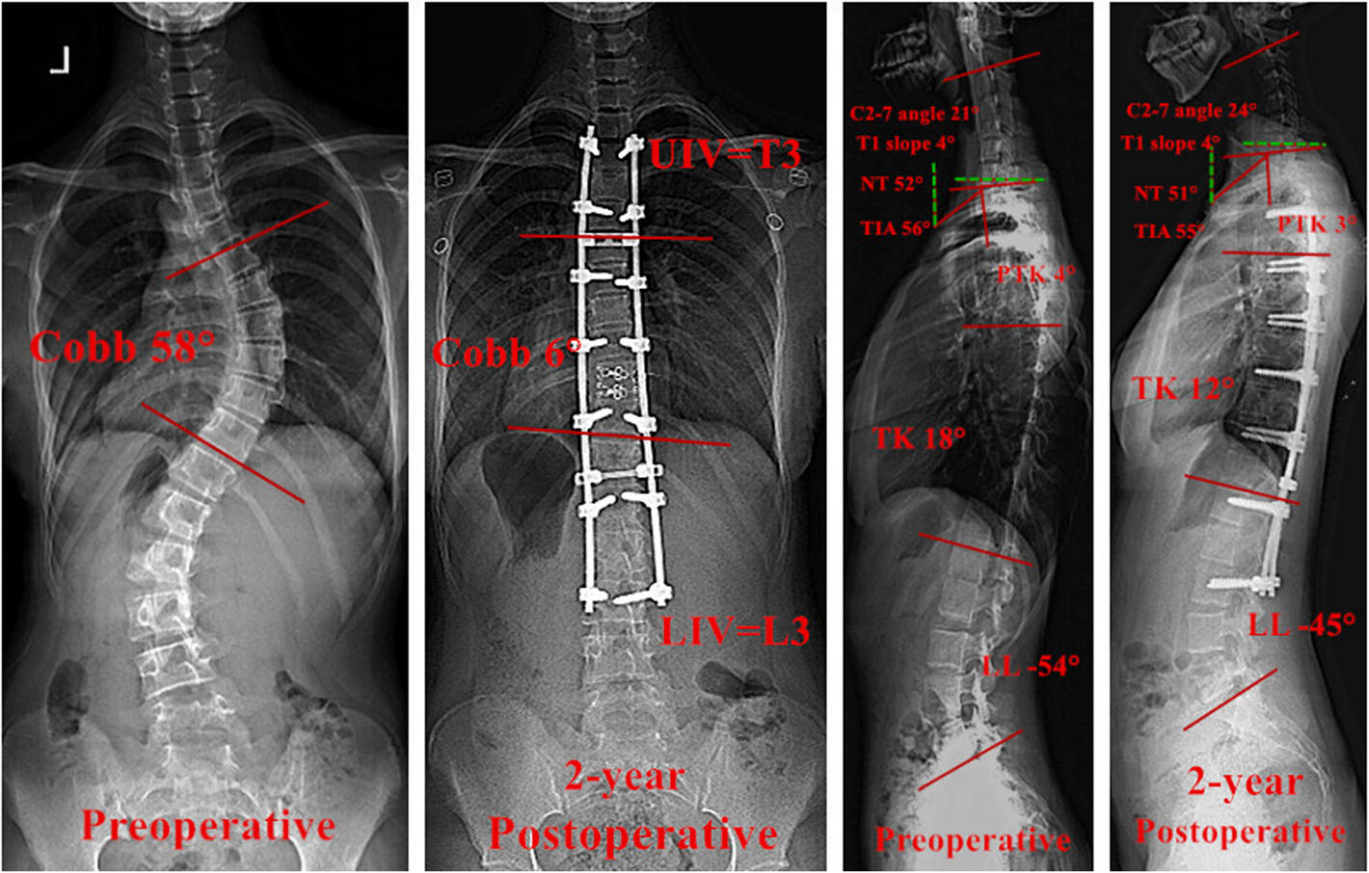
The pre- and 2-year postoperative radiographs of a Lenke 1 patient with AIS who had uncorrected CK after surgery. The coronal Cobb angle of the *main curve* was corrected from 58° preoperative to 6° postoperative (the rate of corrective Cobb angle was 89.7%). The C2-7 angle varied from 21° preoperative to 24° postoperative. The PTK varied from 4° preoperative to 3° postoperative. The TK decreased from 18° preoperative to 12° postoperative. AIS, adolescent idiopathic scoliosis; CK, cervical kyphosis; CL, cervical lordosis; PTK, proximal thoracic kyphosis; TK, thoracic kyphosis
